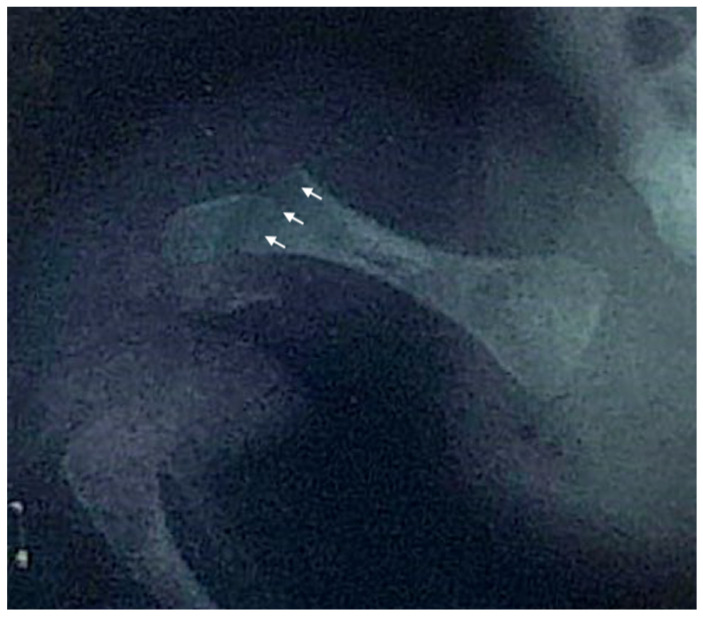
Anteroposterior X-ray of the right hip and proximal femur of the newborn, demonstrating a right femoral fracture. Arrows indicate the fracture line through the distal femoral shaft and associated cortical discontinuity.
